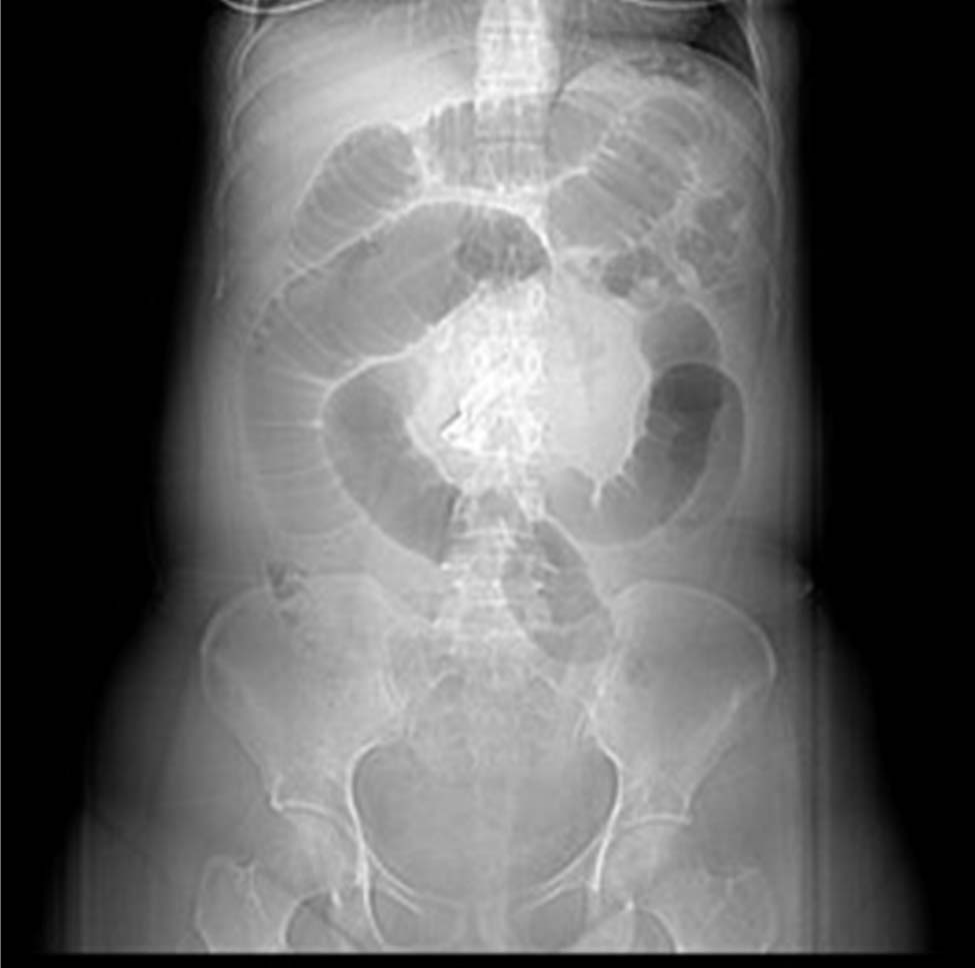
The abdominal radiograph showed dilated small bowel loops.
